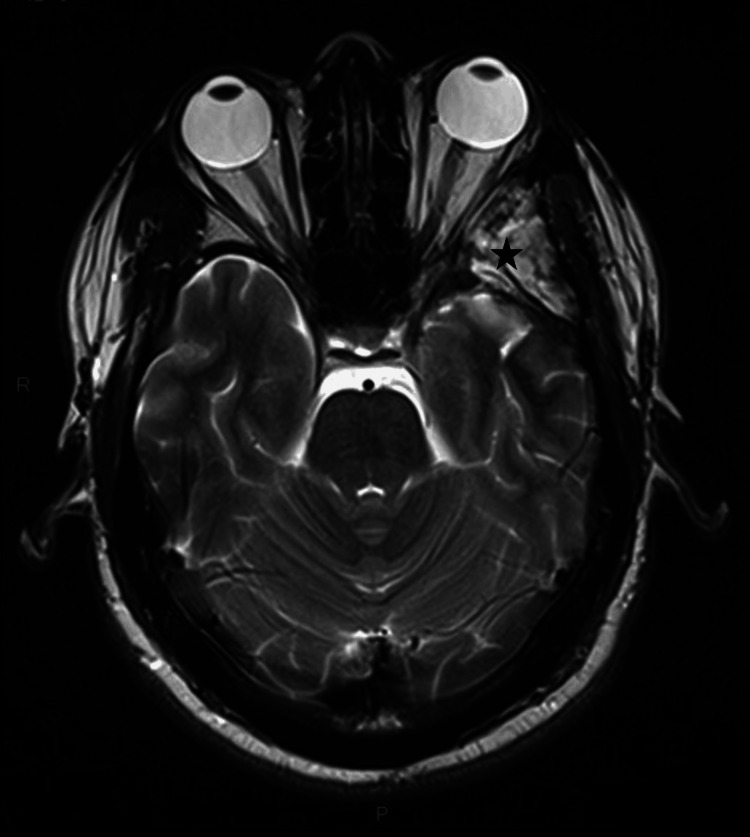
Postoperative axial T2-weighted brain MRI. Complete resection of the different components of the lesion (black star).
